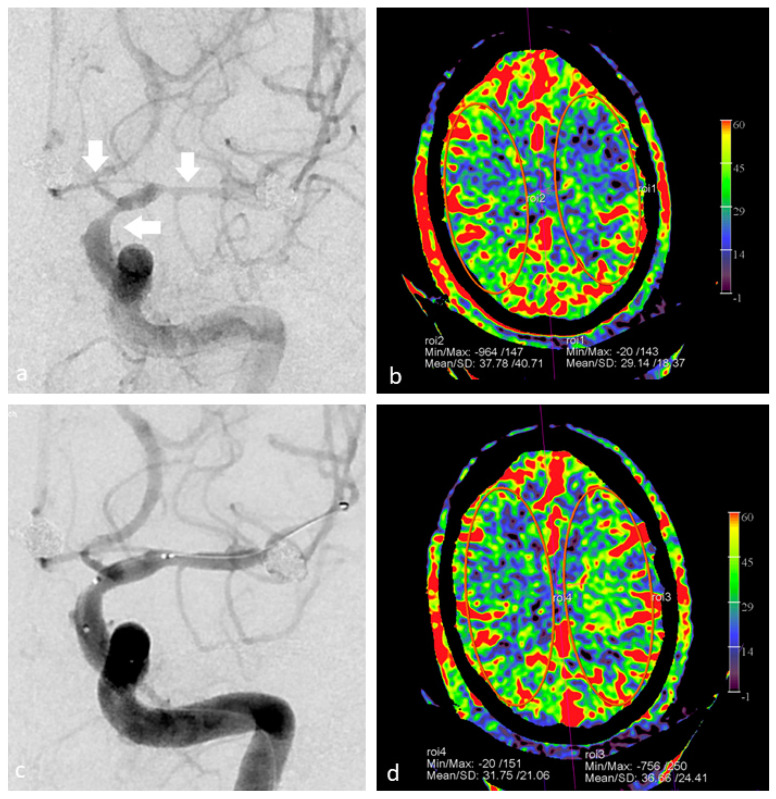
FD CT PBV imaging in a 44-year-old patient after subarachnoid hemorrhage with symptomatic vasospasm. (a) DSA in AP view demonstrates severe vasospasm at the level of the left distal internal carotid artery, proximal anterior and middle cerebral arteries (white arrows). (b) FD CT PBV mapping with PBV measurements before EVT, displaying lower PBV values at the left hemisphere, compared to the right hemisphere. (c) DSA image post dilatation shows favorable angiographic response to PTA. (d) Post PTA FD CT PBV measurements show improvement of the PBV value at the left hemisphere compared to the right hemisphere.
